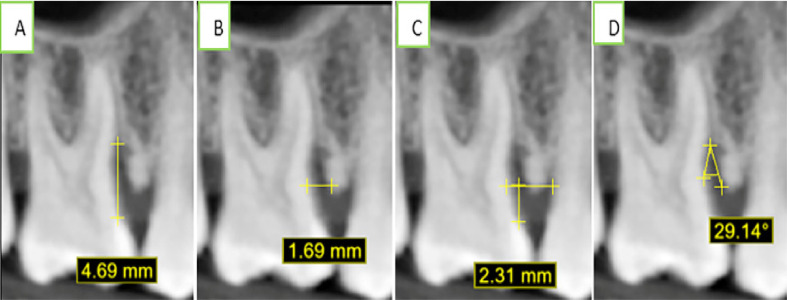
Radiographic measures after 6 months follow up. (A) defect bone fill after 6 months= 4.69 mm; (B) defect width= 1.69 mm; (C) crestal bone formation= 2.31 mm; (D) angle measure= 29.14°
